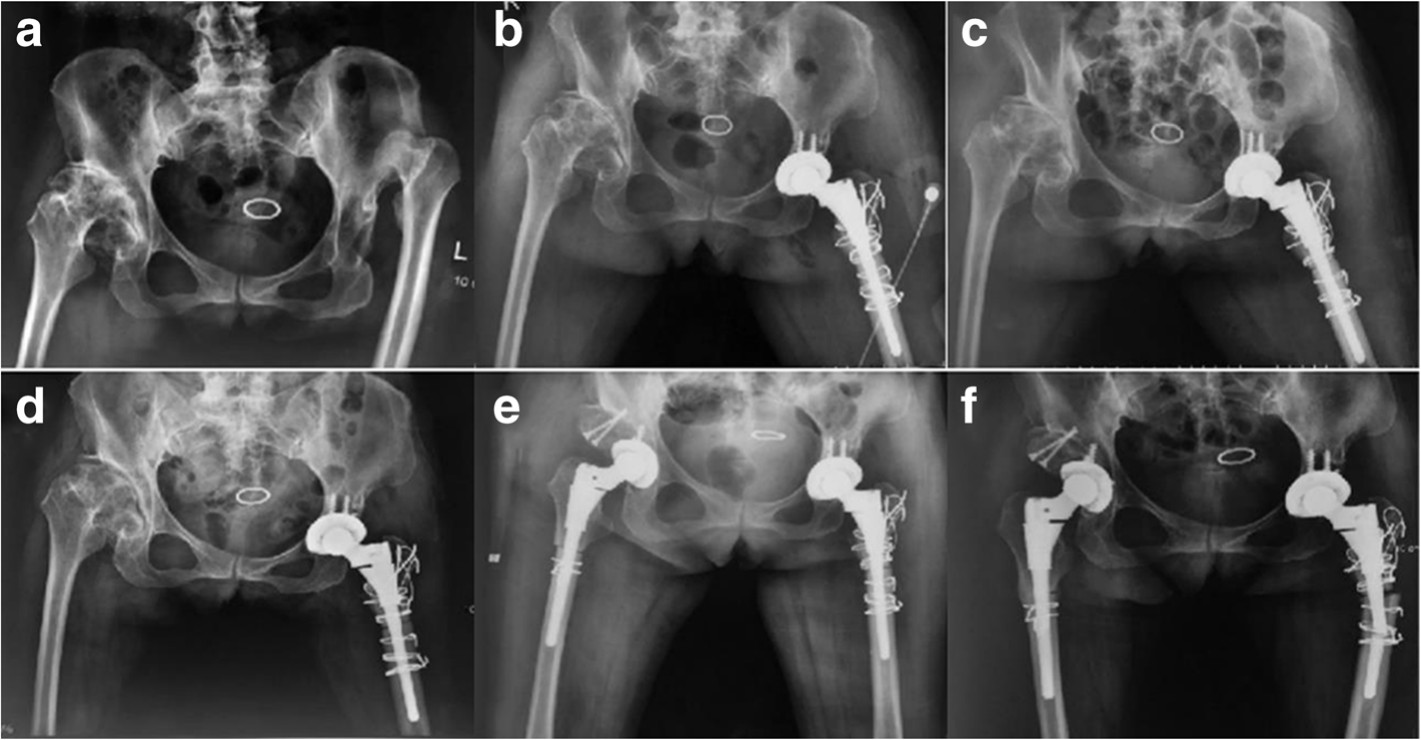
Radiographic images before revision surgery (a-f). a Crowe type IV DDH was found at the left hip before primary surgery. b-e Radiographic images of the patient’s left primary THA 1 day, 1 month, 3 months, 5 months postoperatively. f The radiographic examination of the patient’s left primary THA 14 months postoperatively shows that the distal stem is broken and the subtrochanteric osteotomy of the left femur is not healed
